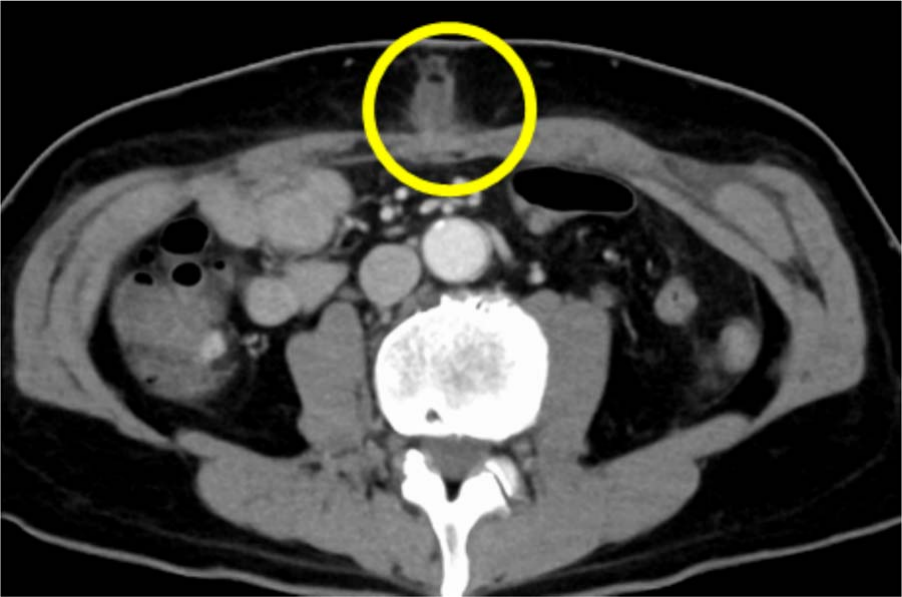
CT findings suggestive of an SSI. The circles indicate abscesses.
